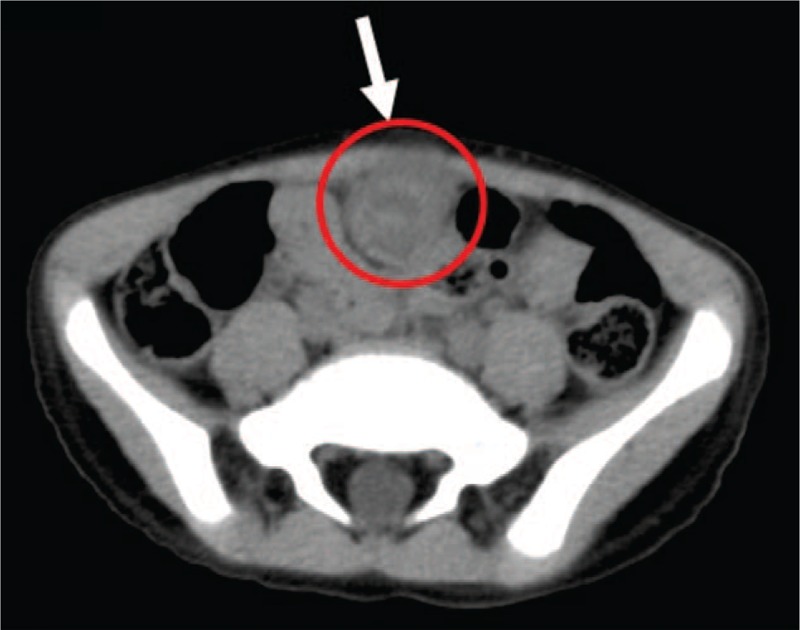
Transverse view of abdominal CT (non-contrast): 2 × 2 cm cystic-like mass lesion (arrow) extending from the upper urinary bladder to the umbilicus with peripheral fat stranding and fluid inside, which suggested an infected urachal cyst. CT = computed tomography.
